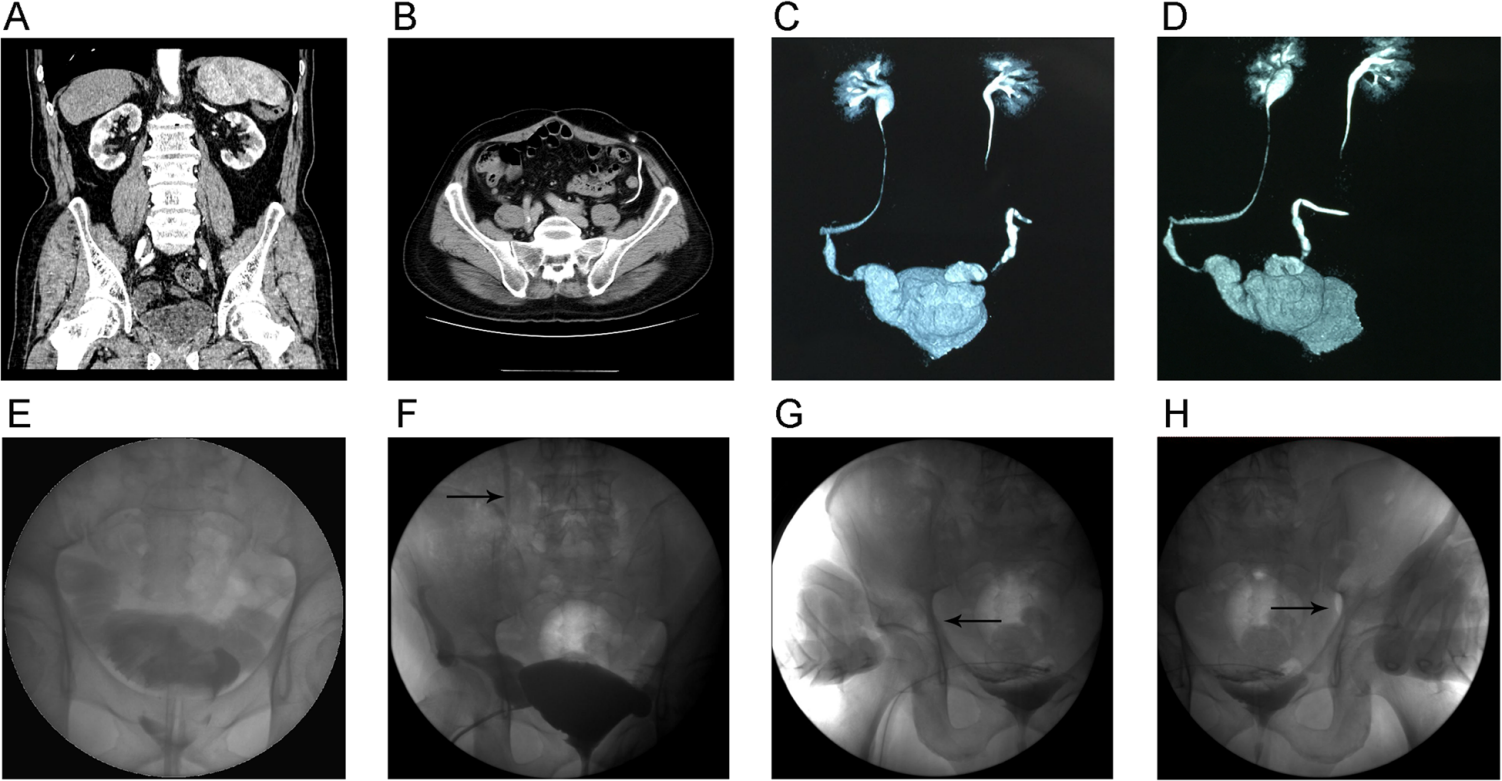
Computed tomography scan of urinary system and cystography data after the operation. a Coronal position of CT. b Horizontal position of CT. c Three-dimensional (3D) enhanced CT (anterior-posterior position). d 3D enhanced CT (lateral position). e Bladder capacity of cystography. f Right side: vesicoureteral reflux during urination of cystography, solid arrow indicating vesicoureteral reflux. g and h Right side and left side: vesicoureteral reflux could be completely prevented by pressing the corresponding point on the abdominal surface when straining for voiding urine; solid arrow indicates the pressure point
